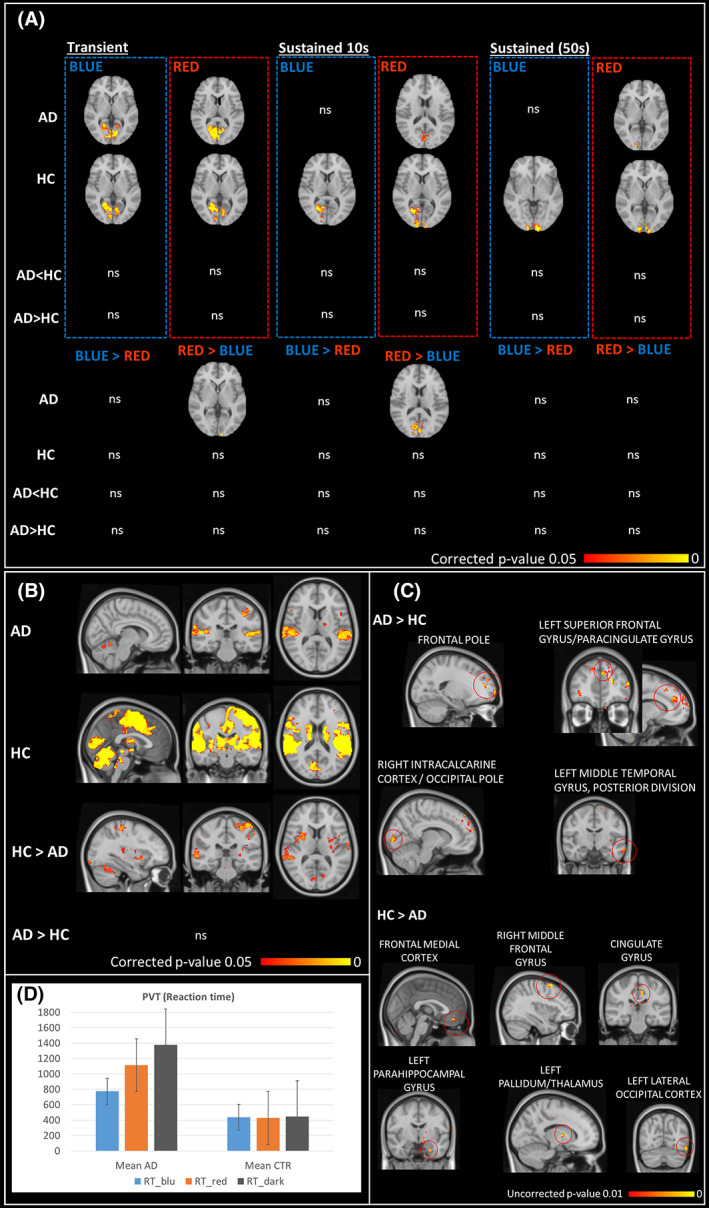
Functional brain MRI activations results. Panel A: functional brain responses to blue and red light stimulations for transient and sustained stimuli. Panel B: brain responses to the cognitive sustained attention task. Panel C: functional brain activations for the comparison between PVT task performed under blue light stimulation compared to PVT performed under red light stimulation (PVTblue > PVTred). Panel D: PVT reaction time under the different light conditions.
